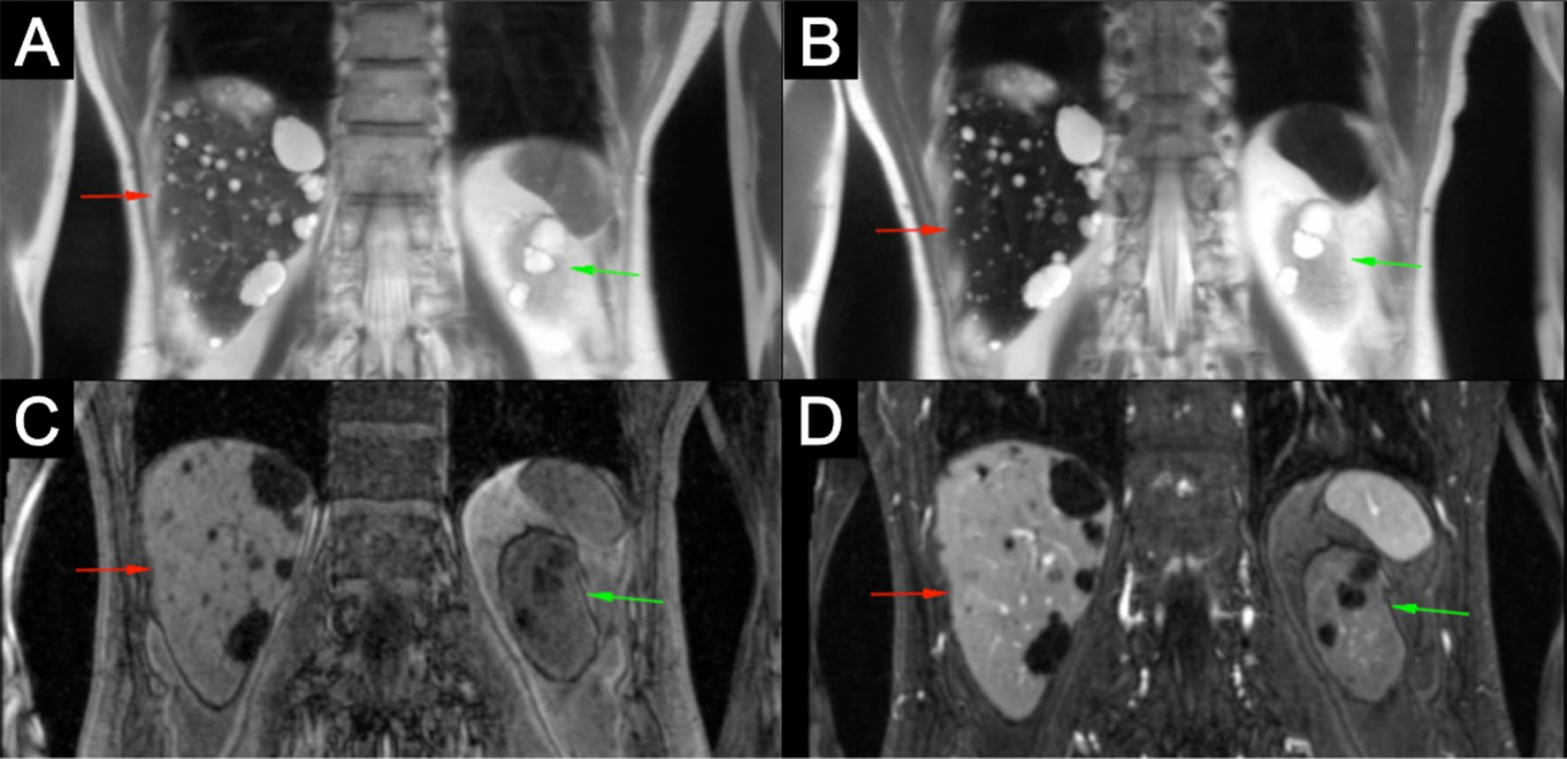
3.0 T ferumoxytol-enhanced MRI of 63-year-old male with polycystic liver and kidneys. Pre-contrast T2 (a) and T1 (c) images show typical cystic appearance with hyperintensity and hypointensity, respectively. Post-contrast images (b and d) reveal increased conspicuity of cysts with administration of ferumoxytol for both hepatic (red arrows) and renal cysts (green arrows), but pronounced improvement for hepatic cysts on T2 images and renal cysts on T1 images
